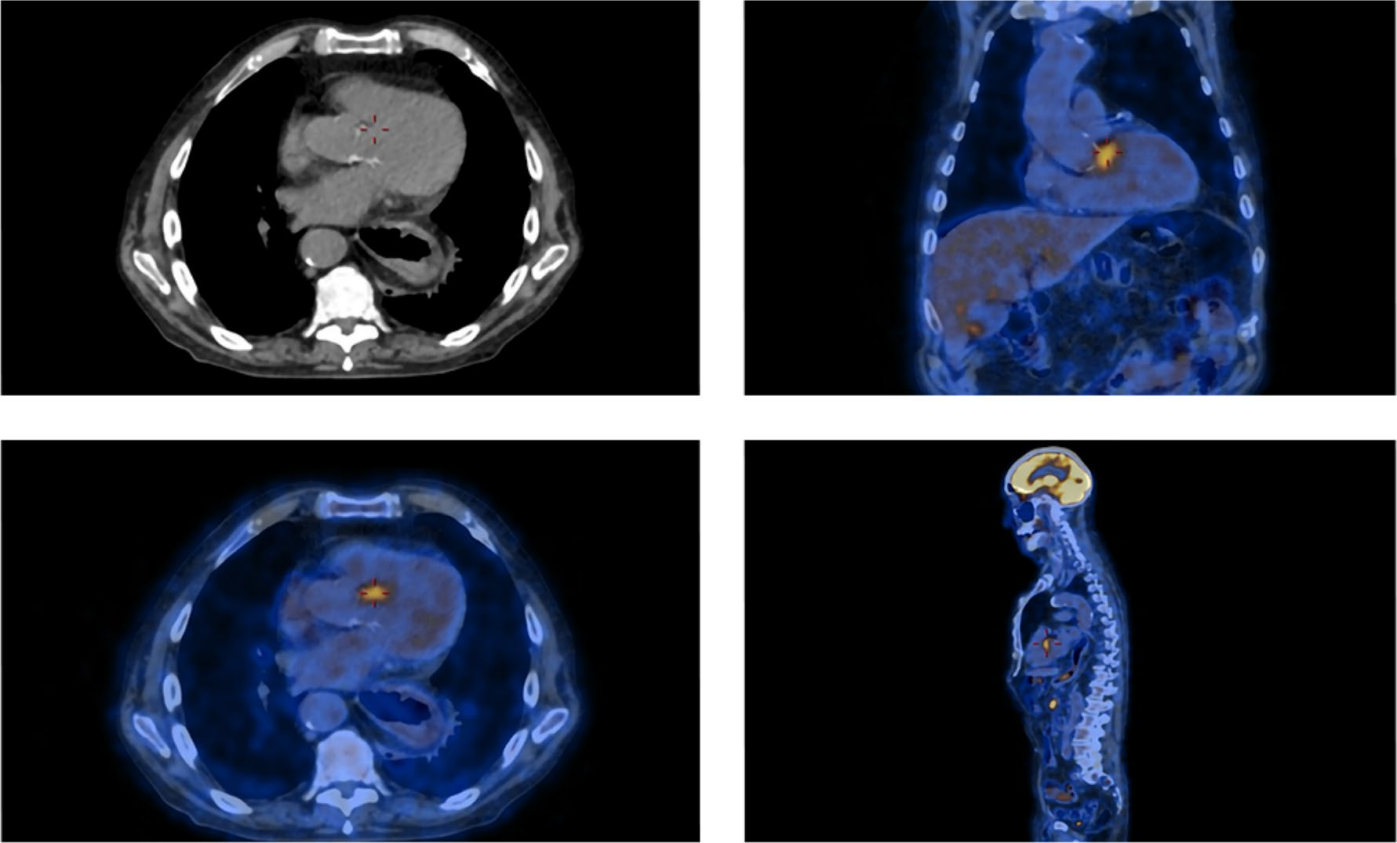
Case 1, 18-FDG–PET/CT scans of aortic valve hypermetabolism. *18-FDG* 18 fluorodeoxyglucose, *PET/CT* positron emitting tomography/computed tomography
